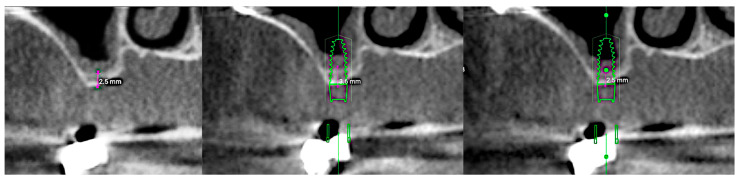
From left to right: preclinical situation (CBCT), implant planning at the first molar site (residual bone height: 3.6 mm), implant planning at the second molar site (RBH 2.5 mm).
